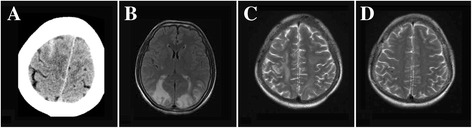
Brain imaging in RCVS. (A) CT scan showing cSAH; (B) FLAIR showing bilateral hypersignals in the occipital lobes consistent with PRES; (C,D) MRI showing right hyperintense subcortical lesions consistent with vasogenic edema and right brain hemorrhage;and cranial MRI showing resolution of the lesion after 12 weeks from the same patient as shown in C.
